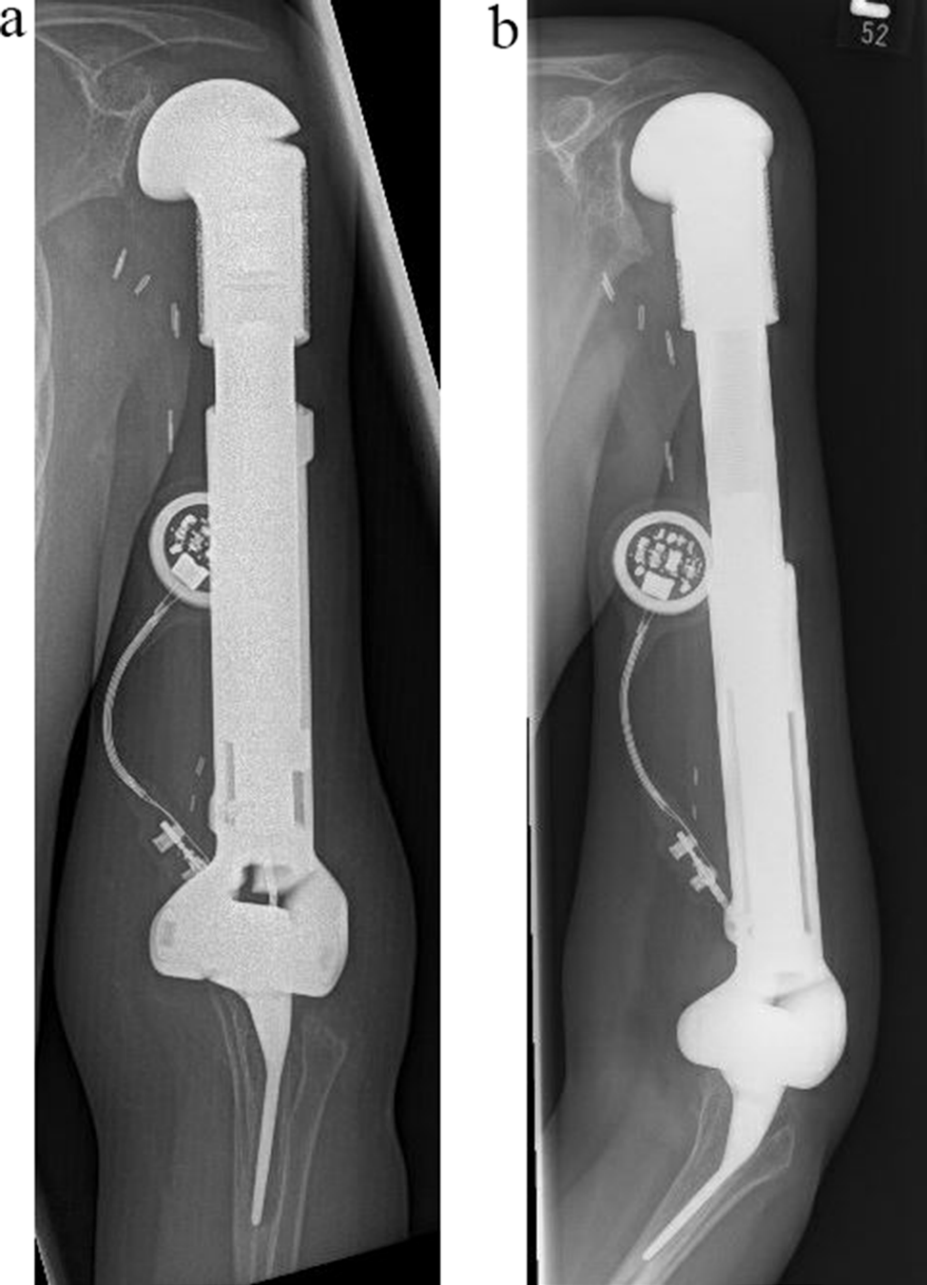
Postoperative radiographs at the beginning (a) and after completion (b) of the elongation process of the Xpand^®^ growing prosthesis (patient #3)
